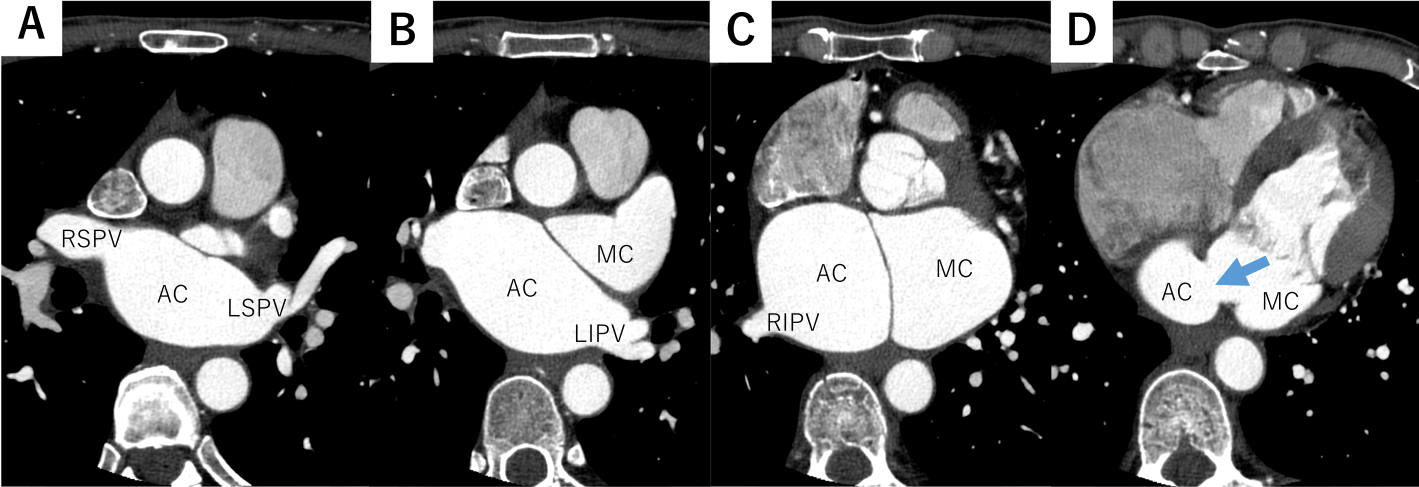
Preoperative enhanced computed tomography. A–C All four pulmonary veins entered the heart through the accessory chamber. D The accessory chamber and the main chamber communicated via an 11-mm hole (blue arrow). RSPV, right superior pulmonary vein; RIPV, right inferior pulmonary vein; LSPV, left superior pulmonary vein; LIPV, left inferior pulmonary vein; AC, accessory chamber; MC, main chamber
